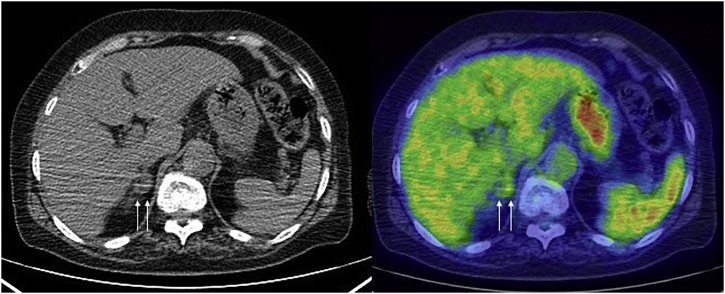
Positron emission tomography–computed tomography scan after chemotherapy. The images show that the right adrenal metastasis decreased in size to 13 mm (arrows).
